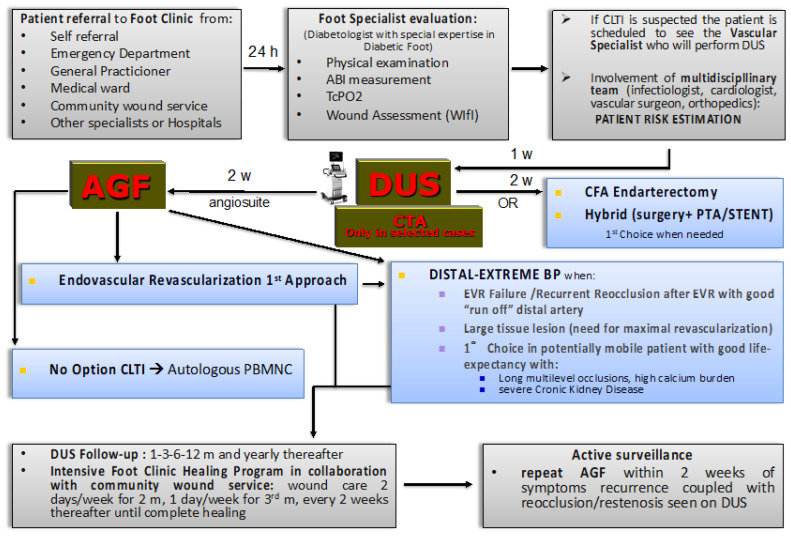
Algorithm of the Arezzo fast-track team-based management of CLTI patients with tissue loss. ABI, ankle-brachial index; TcPO2, transcutaneous oxygen pressure; WIfi, wound, ischemia, foot infection classification; CLTI, chronic limb-threatening ischemia; DUS, duplex ultrasound; AGF, angiography; CTA, computerized tomography angiography; CFA, common femoral artery; PTA, percutaneous transluminal angioplasty; BP, bypass; EVR, endovascular revascularisation; PBMNC, peripheral blood mononuclear cells.
